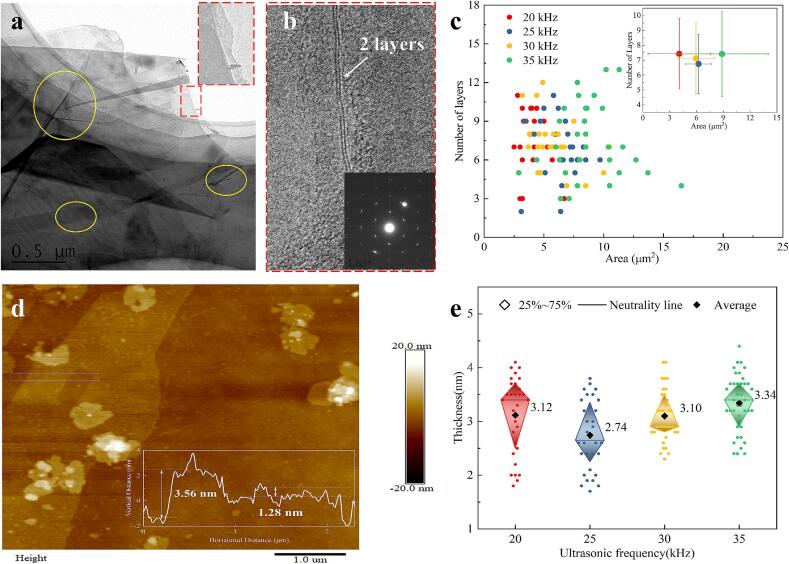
FLG prepared by ultrasonication for 300 min. a: TEM image of FLG prepared at 25 kHz (red dotted line is the local amplification, yellow circle is the crease). b: Local high-resolution TEM images (the lower right corner is the diffraction pattern of the region). c: TEM characterization results. d: AFM image of FLG prepared at 25 kHz. e: AFM characterization results. (For interpretation of the references to colour in this figure legend, the reader is referred to the web version of this article.)
